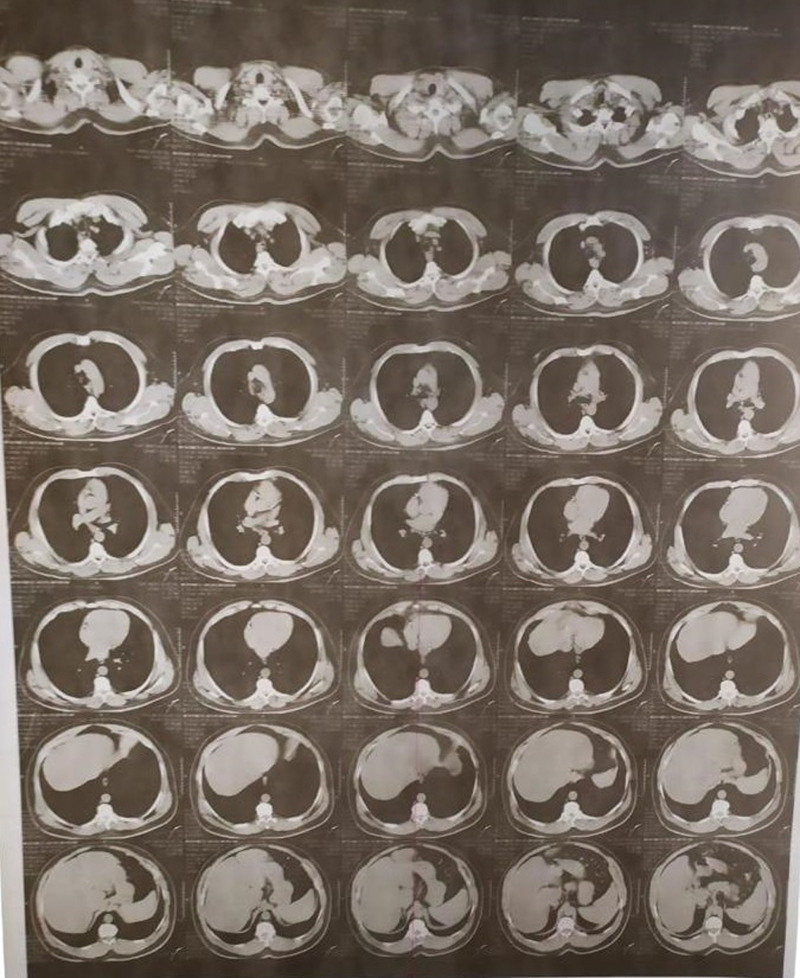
Chest CT of the patient with Takotsubo syndrome.
